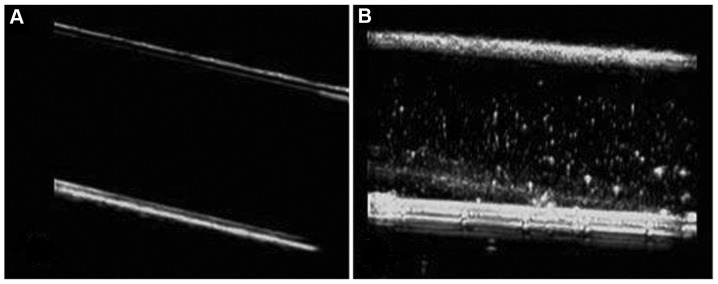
Ultrasound contrast-enhanced images *in vitro*. (A) Tube filled with degassed water; (B) tube filled with paclitaxel-poly(lactic-co-glycolic acid)-monomethoxy poly(ethylene glycol) nanocapsule solution.
